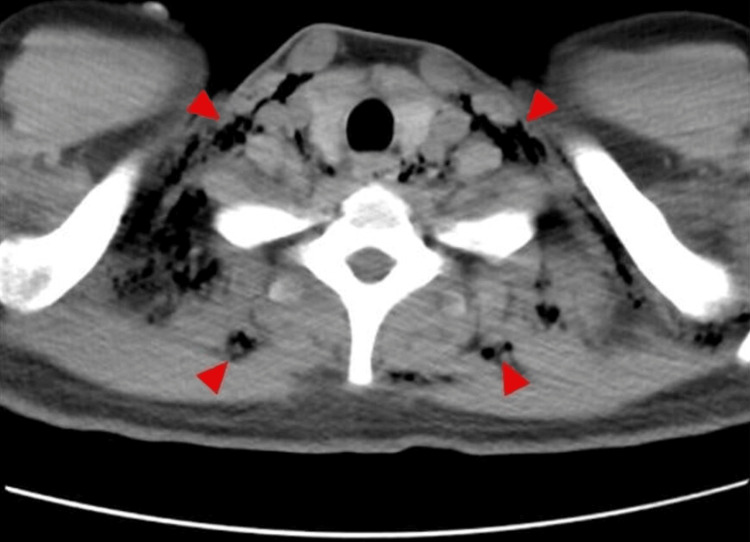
Contrast-enhanced CT showed extensive subcutaneous emphysema from the cervical to mediastinal regions (red arrows).
